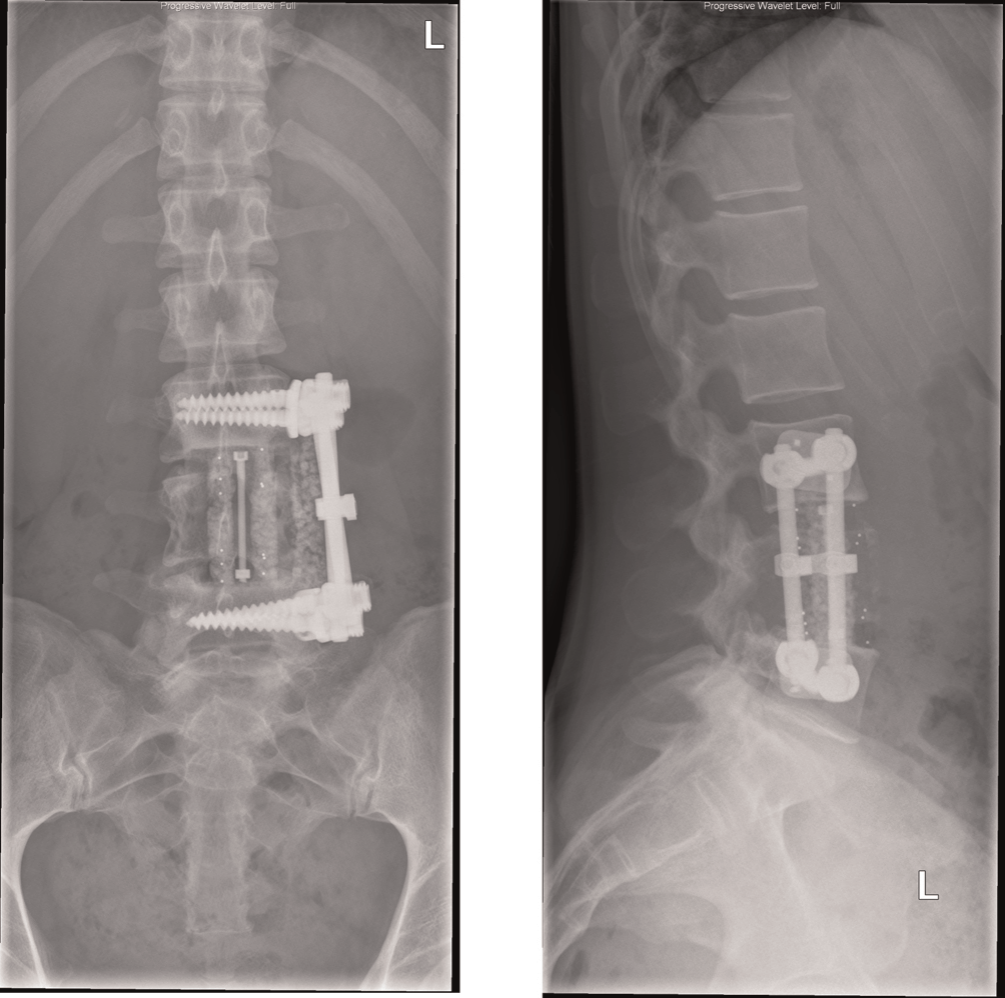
Postoperative AP and lateral radiograph demonstrating the subtotal vertebrectomy of the L4 vertebral body and anterior reconstruction of the defect with the use of an anterior stackable cage and anterior instrumentation.
